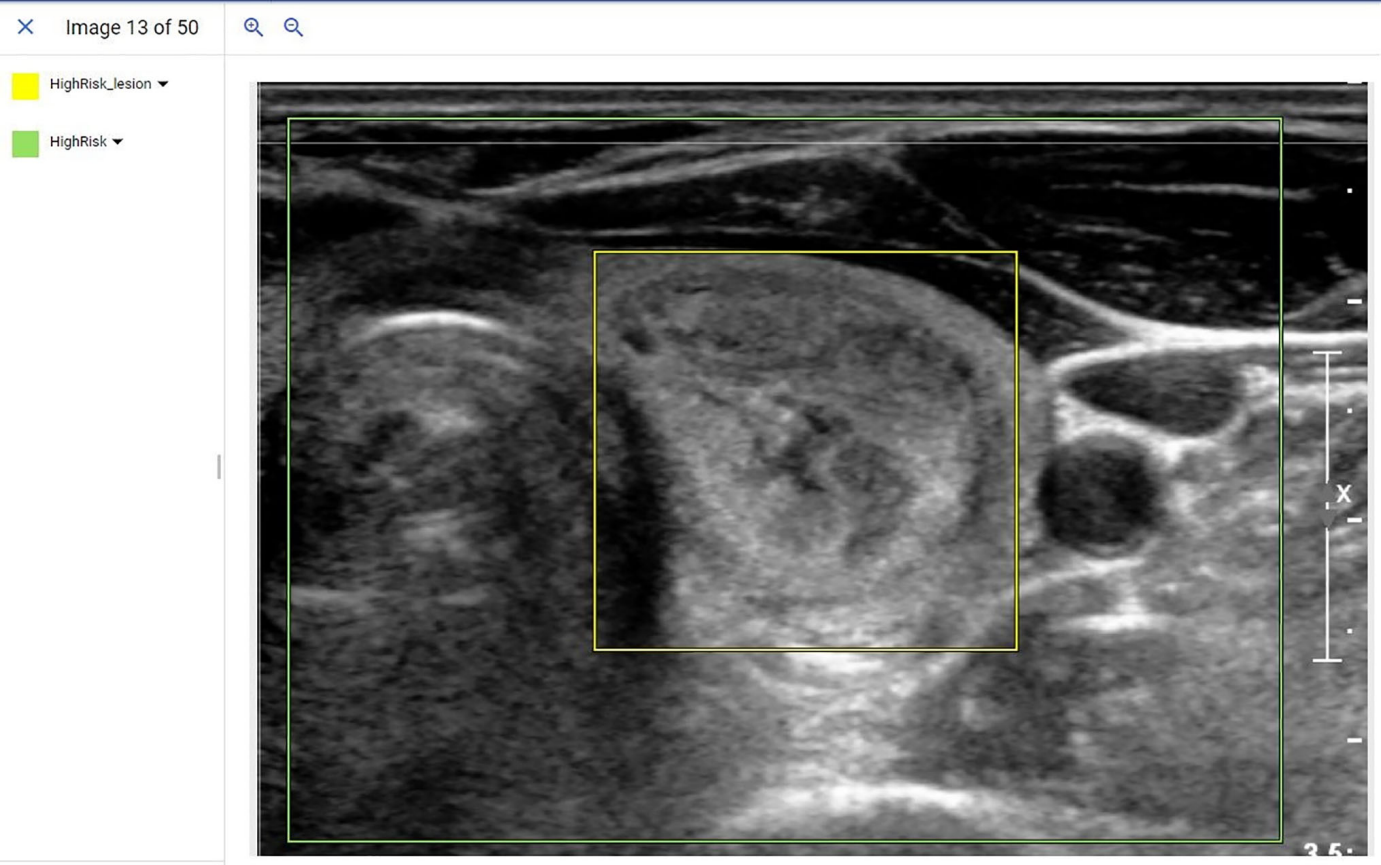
Example of a de-identified image being input into the AutoML object detection model. In this example, radiologists used bounding boxes to mark the location of the lesion (Yellow bounding box) and overall image (Green bounding box). The label was assigned with bounding boxes where the yellow bounding box indicated a high-risk lesion and the green bounding box indicated an ultrasound image that contained a high-risk lesion.
